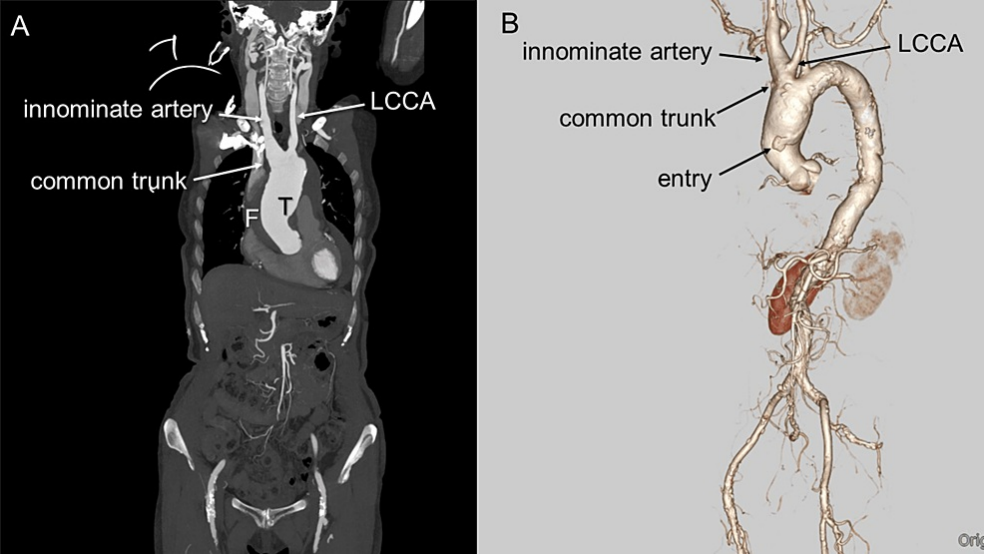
Preoperative CT (A) Coronal CT scan demonstrating a type A acute aortic dissection with the innominate artery and left common carotid artery arising from a common trunk. The dissection is limited to the ascending aorta. (LCCA=left common carotid artery, T=true lumen, F=false lumen) (B) 3D CT demonstrating the entry at the ascending aorta. (LCCA=left common carotid artery)
